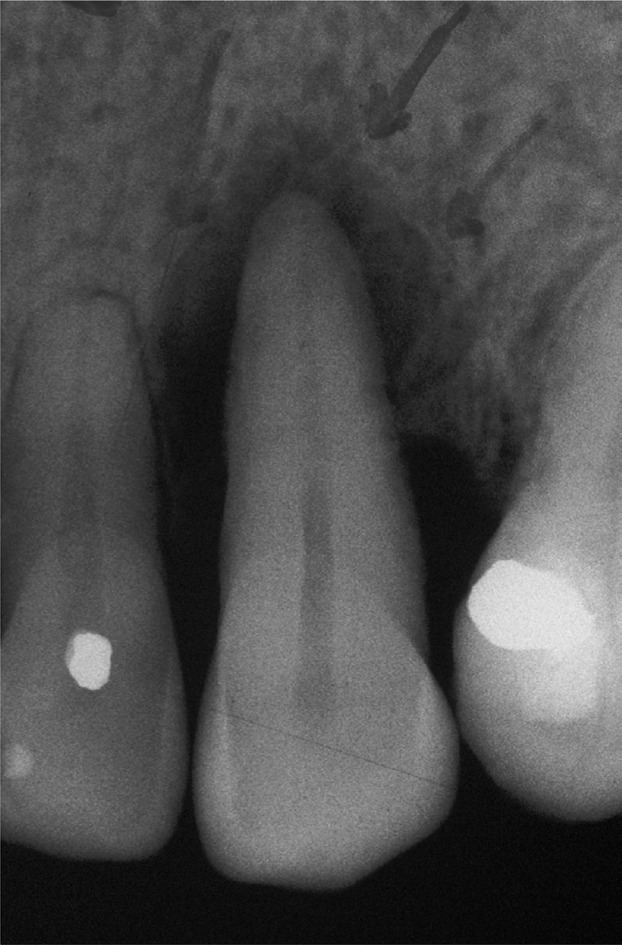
Extensive periapical and lateral radiolucency.
